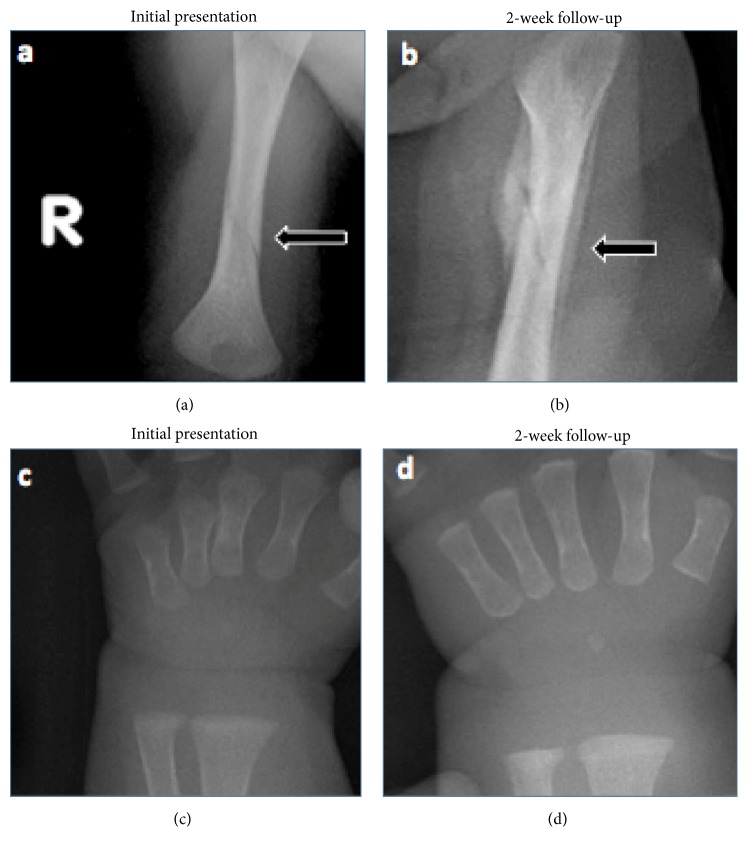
(a) Radiograph of the right upper extremity with obliquely orientated lucency through the distal humeral metadiaphysis with associated soft tissue swelling that is consistent with a nondisplaced spiral fracture. (b) Follow-up radiograph of right upper extremity with ongoing interval healing of the right spiral fracture of the middistal diaphysis of the right humerus with surrounding periosteal new bone/callus formation. (c, d) Radiographs of the right wrist at initial presentation and two-week follow-up with no signs of cupping or fraying.
